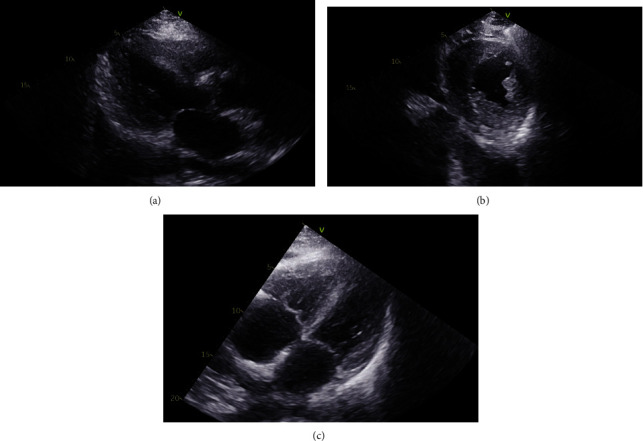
Previous echocardiogram (a). Parasternal long axis (b). Parasternal short axis (c). Apical four-chamber showing moderate concentric left ventricular hypertrophy, right ventricular dilation, and moderately dilated atria.
